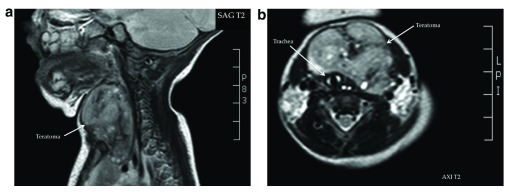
a. Sagittal MRI (T2) of the neck showing the teratoma.
